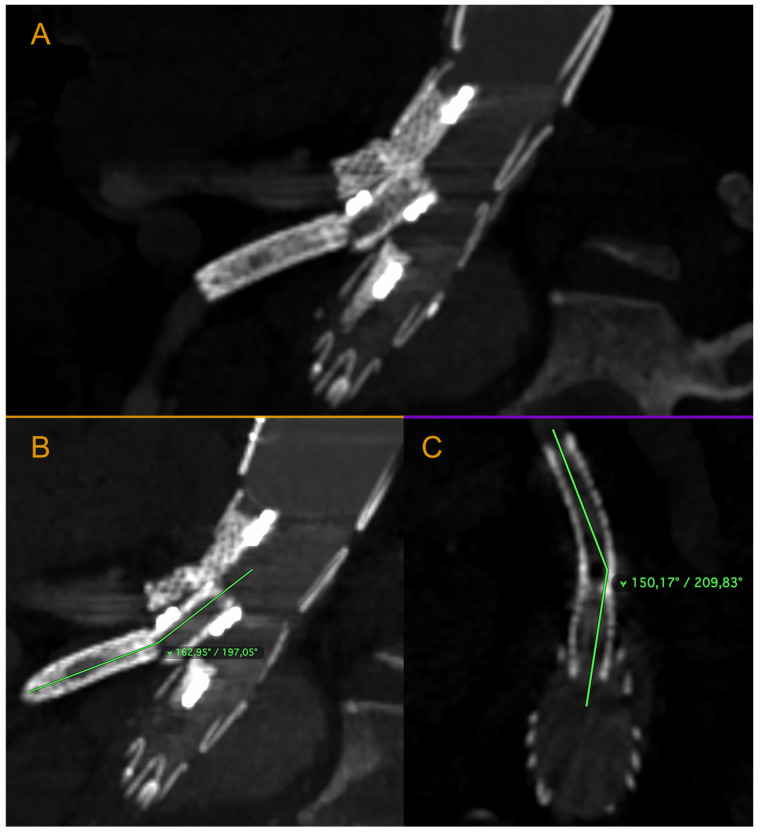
Ruptured superior mesenteric artery branch of stent-graft—the angle between the BeGraft and the vessel. The figure shows a divergence between the axis of the branch and the axis of the superior mesenteric artery. Panel (A)—sagittal projection, panel (B)—sagittal projection with marked angle, panel (C)—axial projection with marked angle. Pre-interventional Angio-CT scans, arterial phase.
